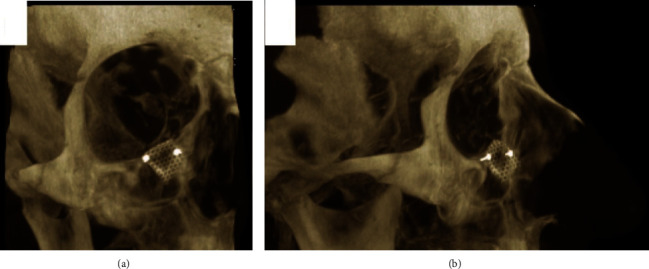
Thick-slice CT sections in the maximum intensity projection (MIP) in (a) frontal and (b) lateral views showing the reconstruction mesh in place.
